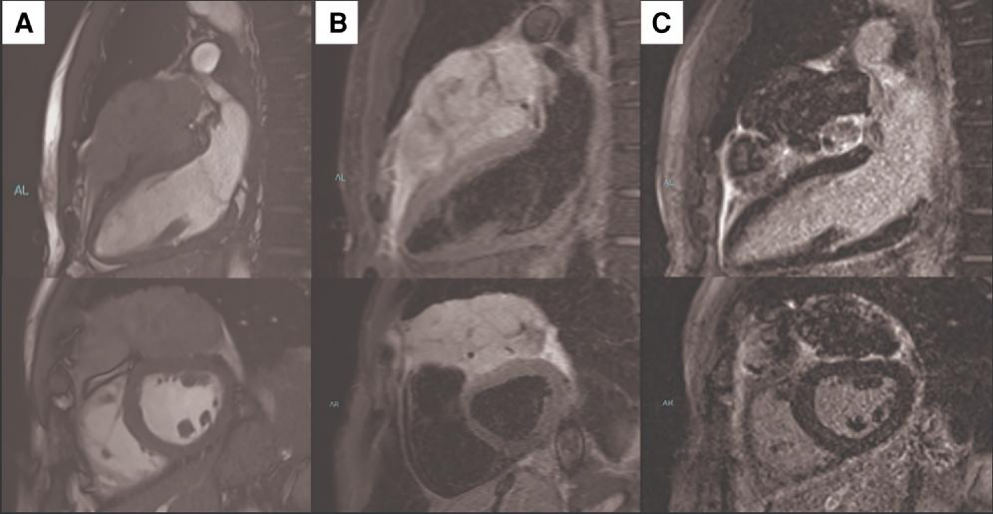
Cardiac magnetic resonance images of the mass. Cine images reveal a large inhomogeneous slightly hypointense mass within the two pericardial layers (*A*). Black-blood T2w images revealed a hyperintensity of the mass and of the pericardial layers (*B*). Late gadolinium enhanced images show a heterogeneous contrast enhancement of the mass and of the pericardial layers (*C*). The mass does not appear to infiltrate the myocardium.
